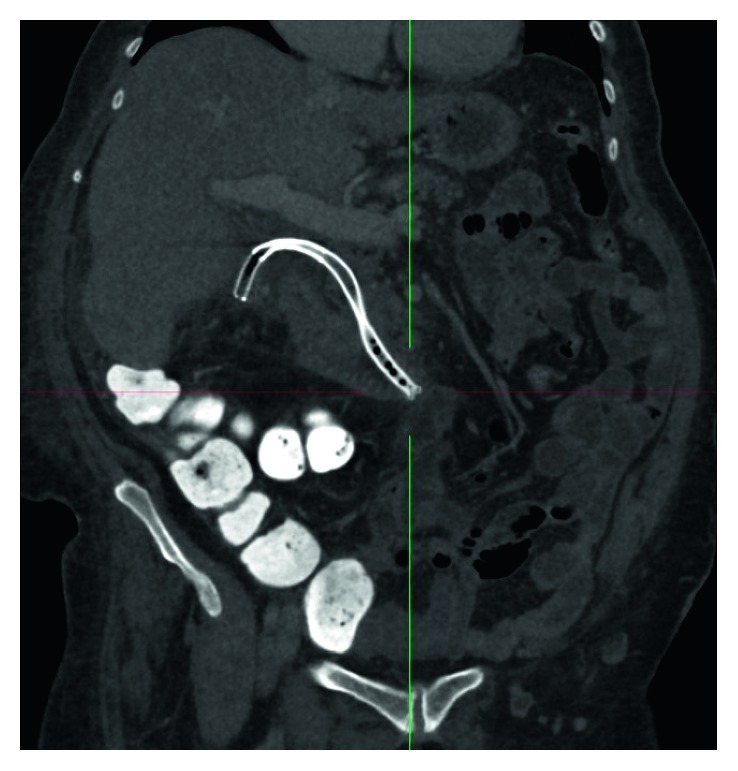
CT scan two days after stent insertion demonstrating appropriate positioning and relative size of the bare metal stents in the cystic and common bile ducts.
